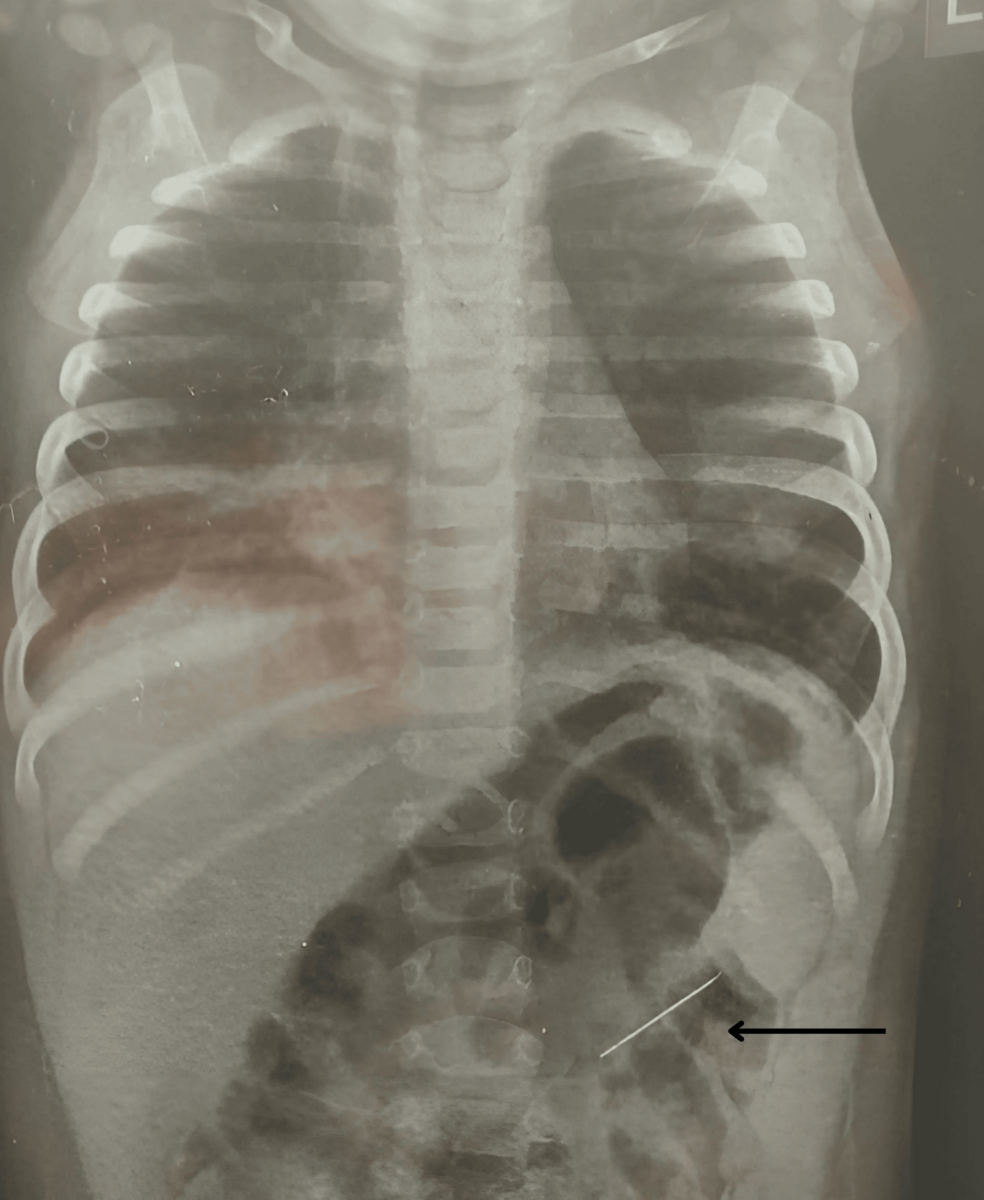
X-ray showing a radio-opaque linear foreign body in the left lumbar region.
